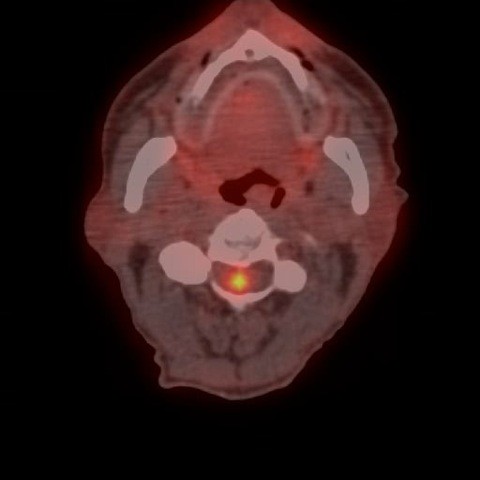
Axial fused PET-CT scan of the cervical spine showing a small focus of intense tracer uptake in the spinal canal at C2-C3 level in the neck.
